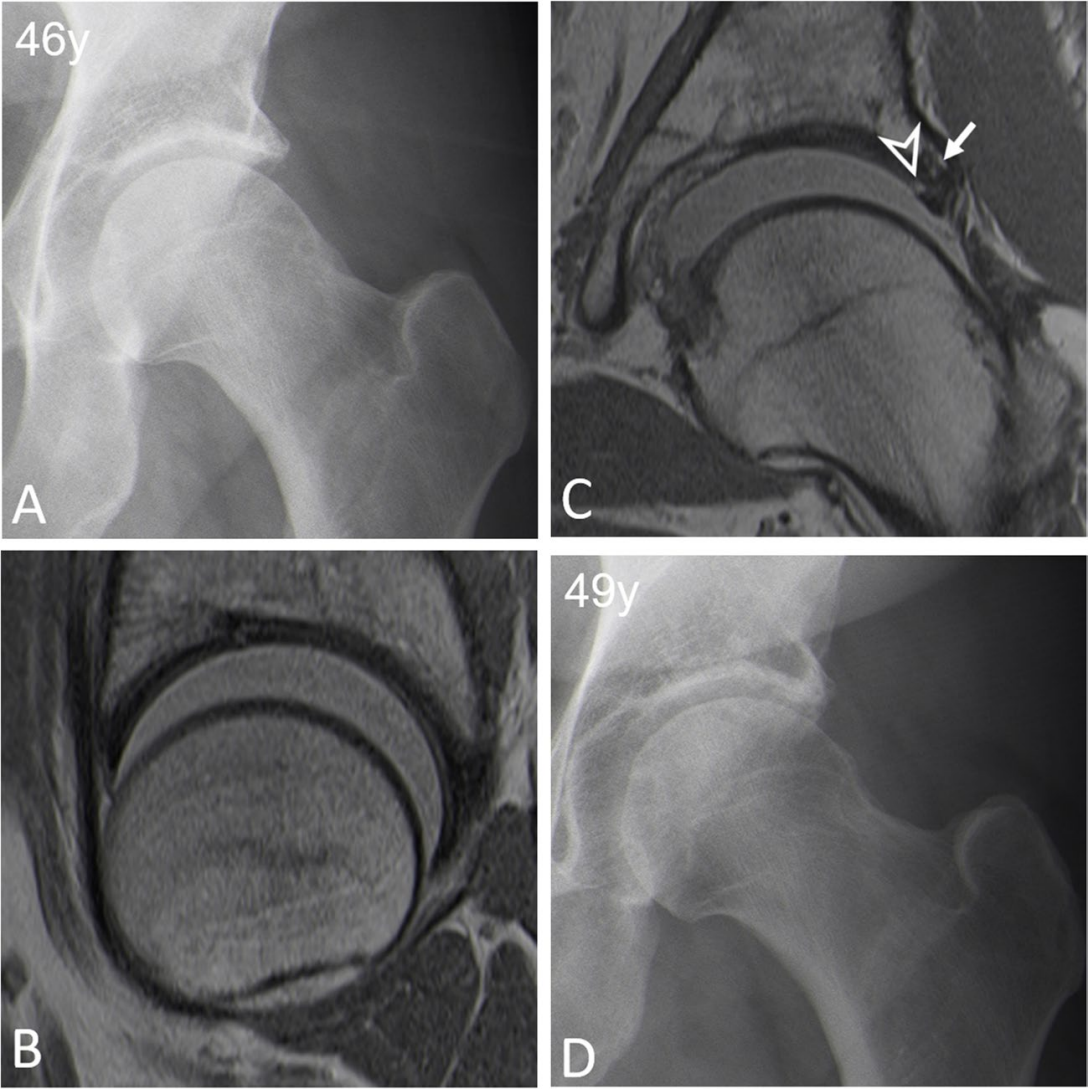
A AP pelvis view of a 46-year-old woman with mixed FAI. B, C Preoperative traction MR-arthrogram with proton density-weighted turbo spin echo images showing none of the MRI findings independently associated with failure of FAI surgery. C On the coronal image, only a focal acetabular cartilage lesion and (arrowhead) and peripheral acetabular osteophyte (arrow) are seen superiorly while the more anterior regions do not show any degenerative findings on the sagittal image (B). D AP pelvis view three years after arthroscopic femoral- and acetabular osteochondroplasty shows a preserved hip joint
